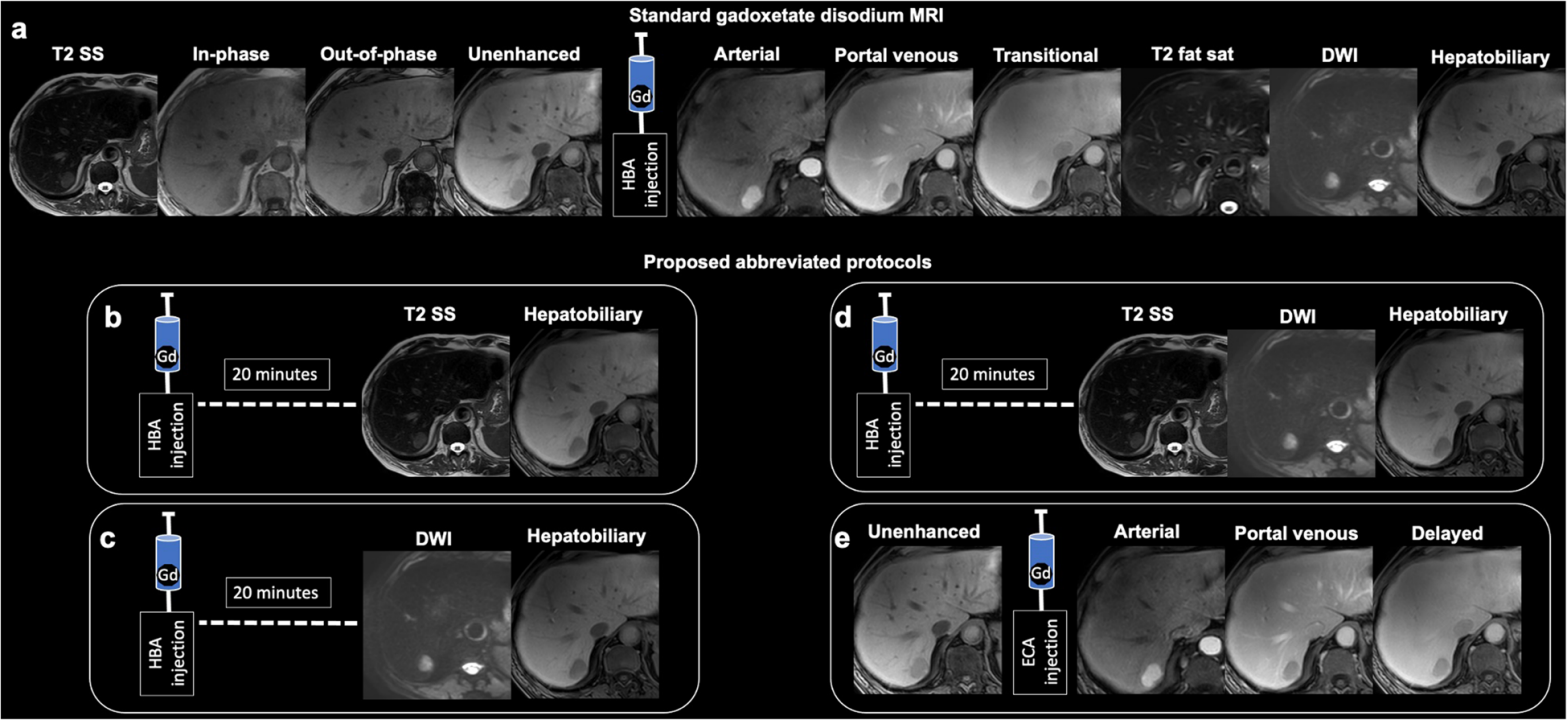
Magnetic resonance imaging of the liver. Standard and proposed abbreviated protocols. 66-year-old man with 30-mm hepatocellular carcinoma (HCC) imaged with standard gadoxetate disodium protocol (a) and proposed abbreviated protocols for HCC screening. Gadoxetate disodium may be administered outside the scanner 20 min before abbreviated protocols that contain a hepatobiliary phase (b-d) or an extracellular agent can be administered before dynamic phases (e). Abbreviated protocols may include T2-weighted single-shot (SS) and hepatobiliary phase (HBP) (b); T2-weighted SS, diffusion-weighted imaging (DWI), and HBP (c); DWI and HBP (d); or pre-contrast and arterial, portal venous, and delayed phases (e). ECA, extracellular contrast agent; HBA, hepatobiliary contrast agent
